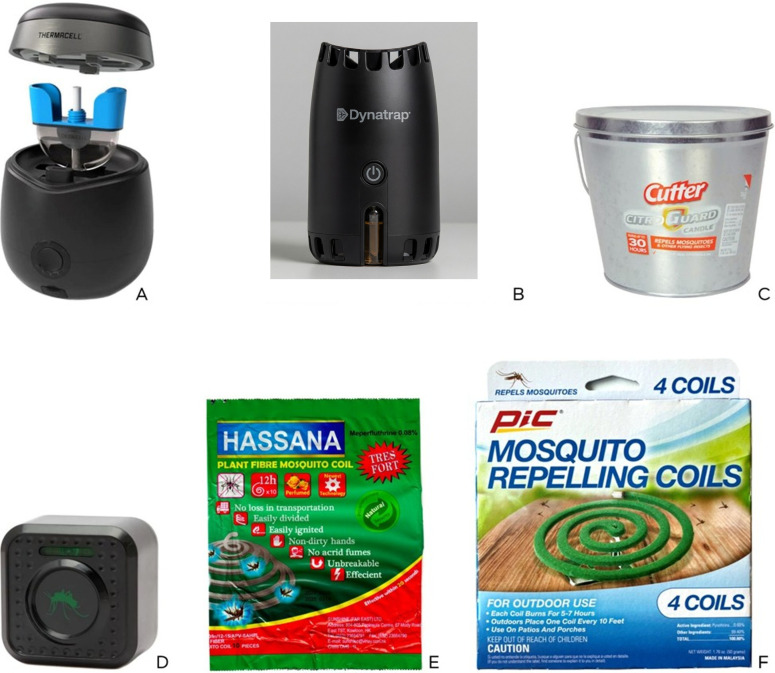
Six commercially available spatial repellents tested in the study. (A) Thermacell^®^ E90 Rechargeable Mosquito Repeller electronic device with a 5.5% transfluthrin-containing replaceable cartridge. (B) Dynatrap^®®^ Mosquito Repellent electronic device with an 8.83% transfluthrin-containing replaceable heat-activated cartridge. (C) Cutter^®^ CitroGuard^®^ Candle containing 3% citronella oil. (D) Isotronic^®^ mosquito repellent “eye” device with an oscillating frequency technology (ultrasonic). (E) Hassana mosquito coil containing 0.08% meperfluthrin. (F) PIC^®^ mosquito repelling coil containing 0.6% pyrethrins.
